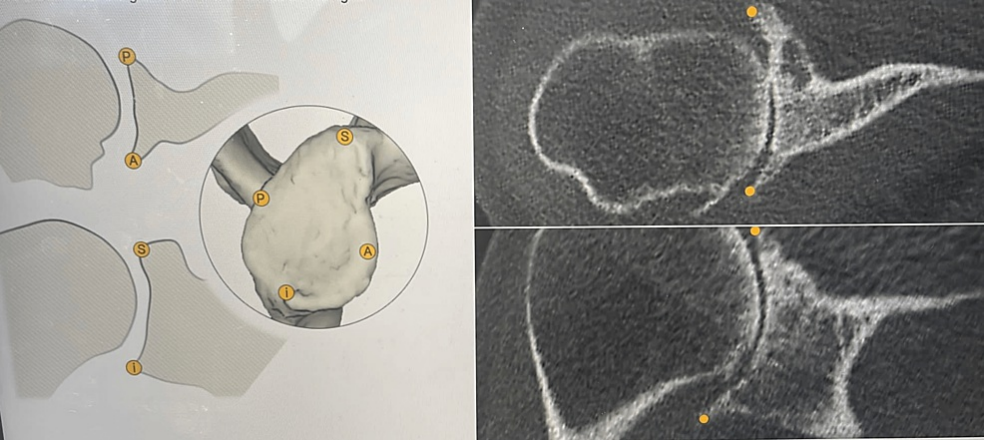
Preoperative planning software (Equinoxe planning software, Exactech BlueOrtho): three-dimensional analysis of the glenoid morphology by assessing the glenoid margins. A: Anterior border of the glenoid. P: Posterior border of the glenoid. S: Superior margin of the glenoid, I: Inferior margin of the glenoid. Yellow circles: Anterior-posterior and superior-inferior margins of the glenoid (axial and coronal planes of computed tomography).
